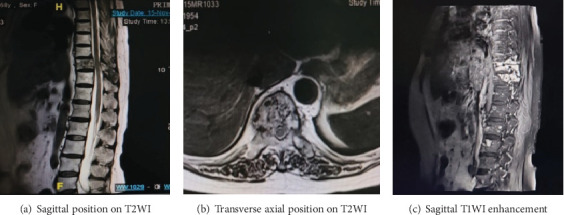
MRI imaging from November 2023. (a) Sagittal position on T2WI indicated uneven signals in the T10 vertebral body and local spinal stenosis, which had improved. (b) Transverse position on T2WI indicated uneven signals in the T10 vertebral body and accessories and clear signals in local bone. (c) Sagittal T1WI enhancement indicated multiple enhancement foci in the thoracolumbar vertebra and the adnexa, narrowing the scope of the anterior lesion.
